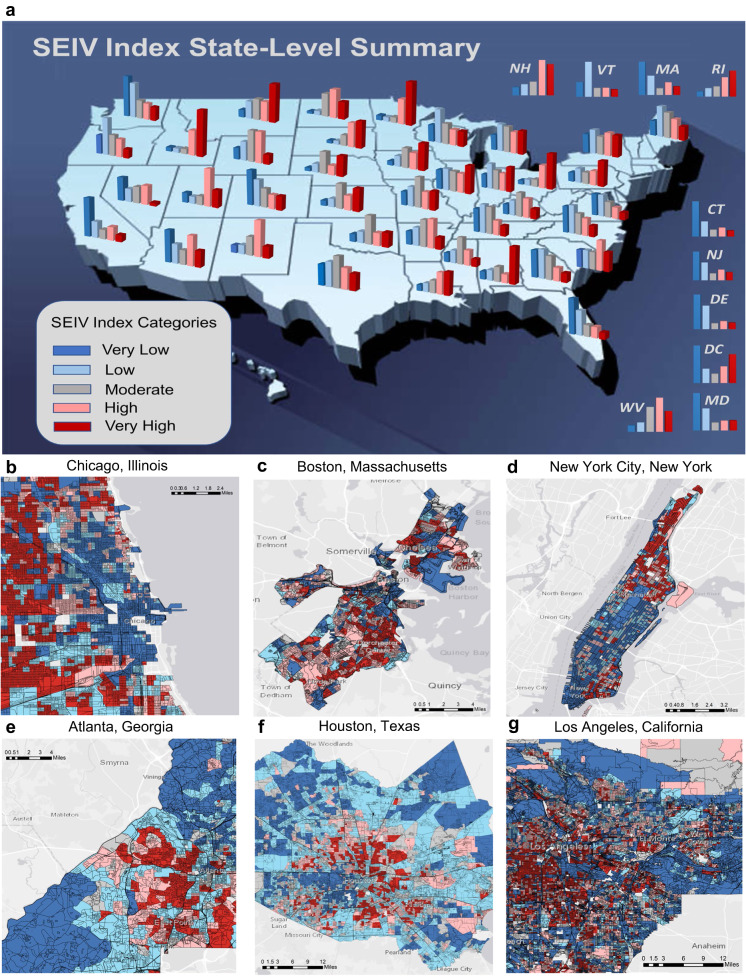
Spatial distribution of Socio-Economic-Infrastructure Vulnerability (SEIV) index statistics over the conterminous United States (CONUS). The upper panel (a) depicts the distribution of SEIV at an aggregate level (here, state level). The lower subpanels (b–g) represent the block-level distribution of SEIV in six large metros of the United States. NH New Hampshire, VT Vermont, MA Massachusetts, RI Rhode Island, CT Connecticut, NJ New Jersey, DE Delaware, DC District of Columbia, MD Maryland, WV West Virginia.
